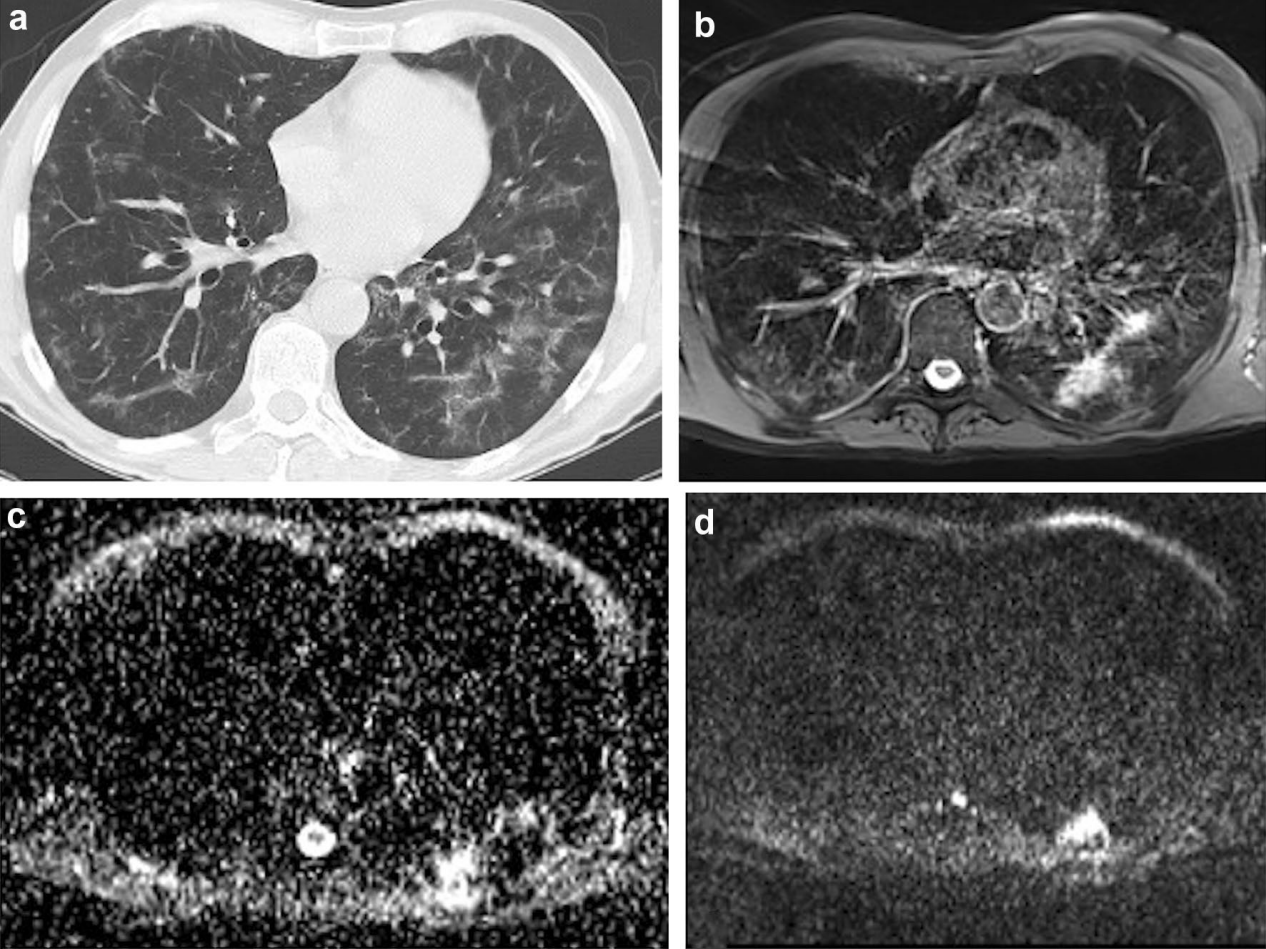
Ground-glass opacities with positive DWI. 58-year-old male. a End-inspiratory axial CT, b Free-breathing PD-weighted axial image, c Apparent Diffusion Coefficient Map, and d) DWI free breathing (*b* = 1000 s/mm^2^). Note an area of GGO on the left lower lobe that shows restricted diffusion on DWI and hyperintensity on ADC map, corresponding to an area of acute inflammation
